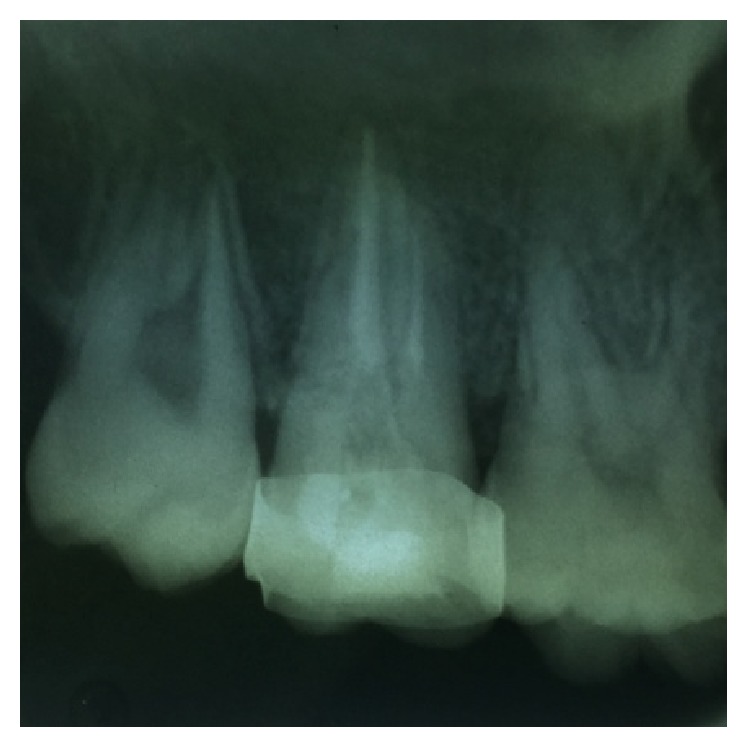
Radiograph showing internal reinforcement with Ribbond in 27.
